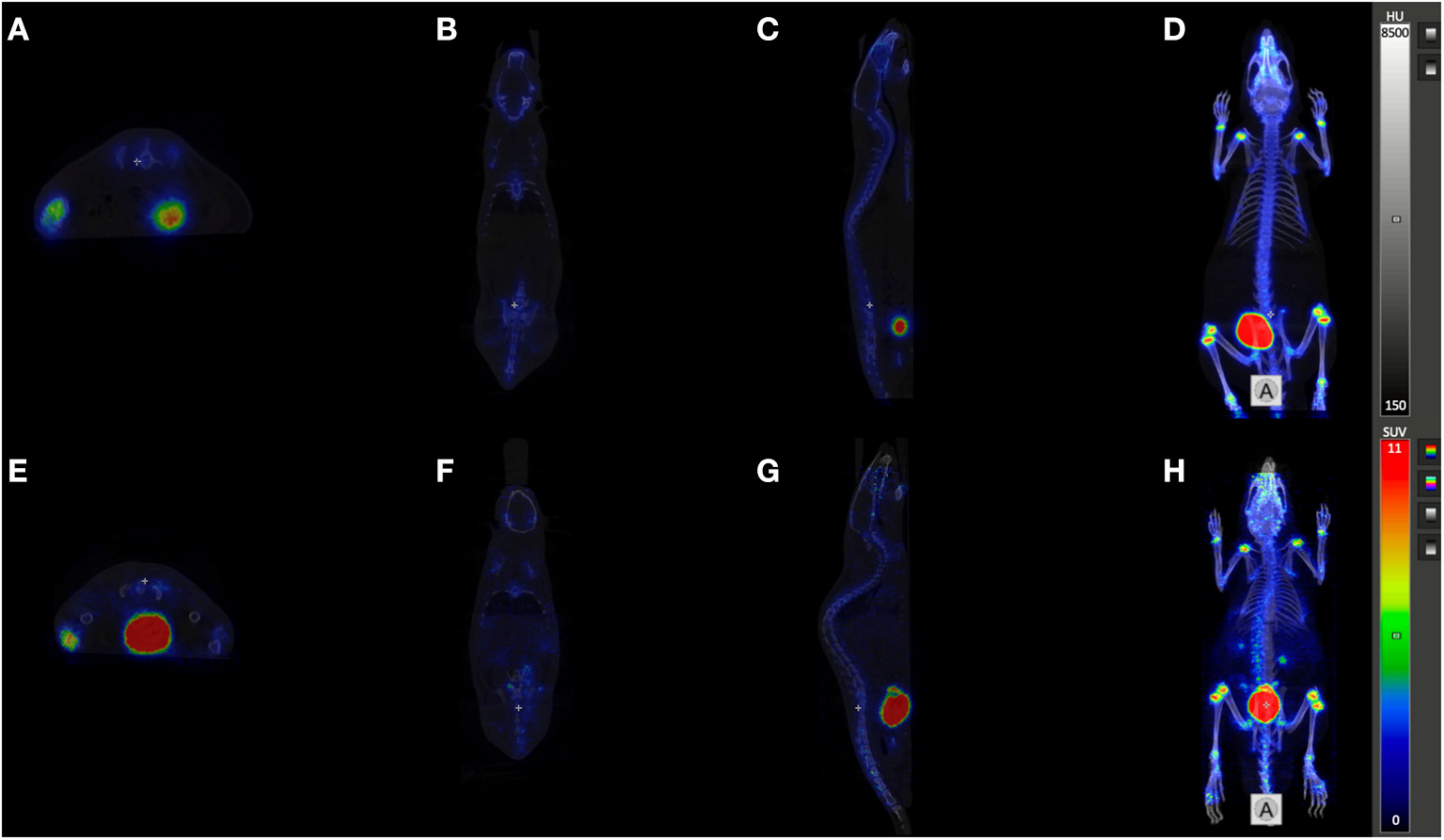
PET/CT imaging: Orthogonal sections (A), E transaxial, (B), F coronal, (C), G sagittal, and Maximum Intensity Projections (D, H) of registered PET/CT studies (midframe time 90 min p.i.) of [^68^Ga]Ga-NODAGA.SA.PAM (A–D) and [^68^Ga]Ga-DOTA-ZOL (E–H).
